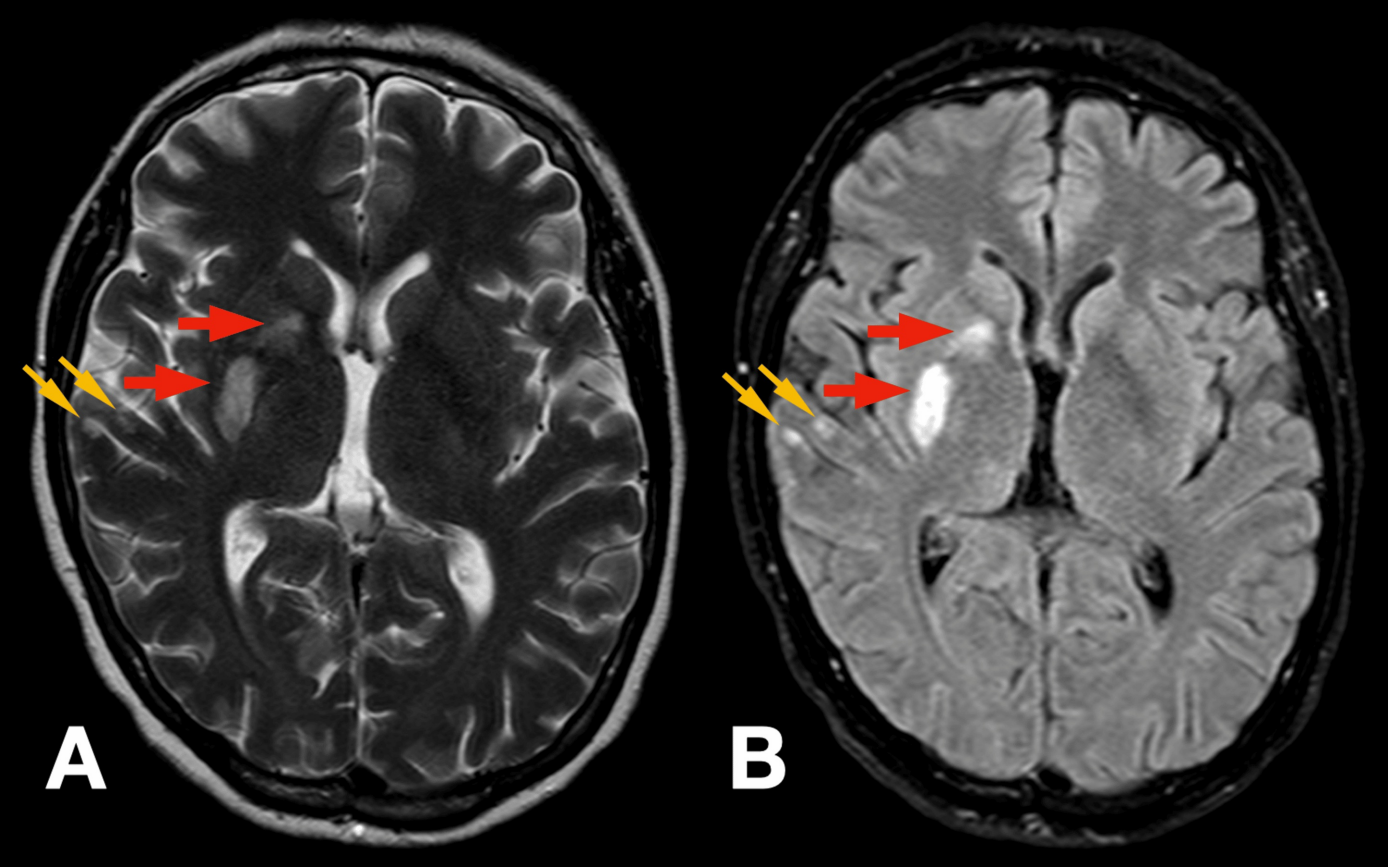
Cranial MRI demonstrating multi-focal ischemic stroke T2-weighted image (Panel A) and fluid-attenuated inversion recovery (FLAIR) image (Panel B) demonstrate hyperintensity in the corona radiata and striatocapsular area (red arrows) and multiple cortical foci within the territory of the right middle cerebral artery (orange arrows) as a result of irreversible acute ischemic brain damage.
